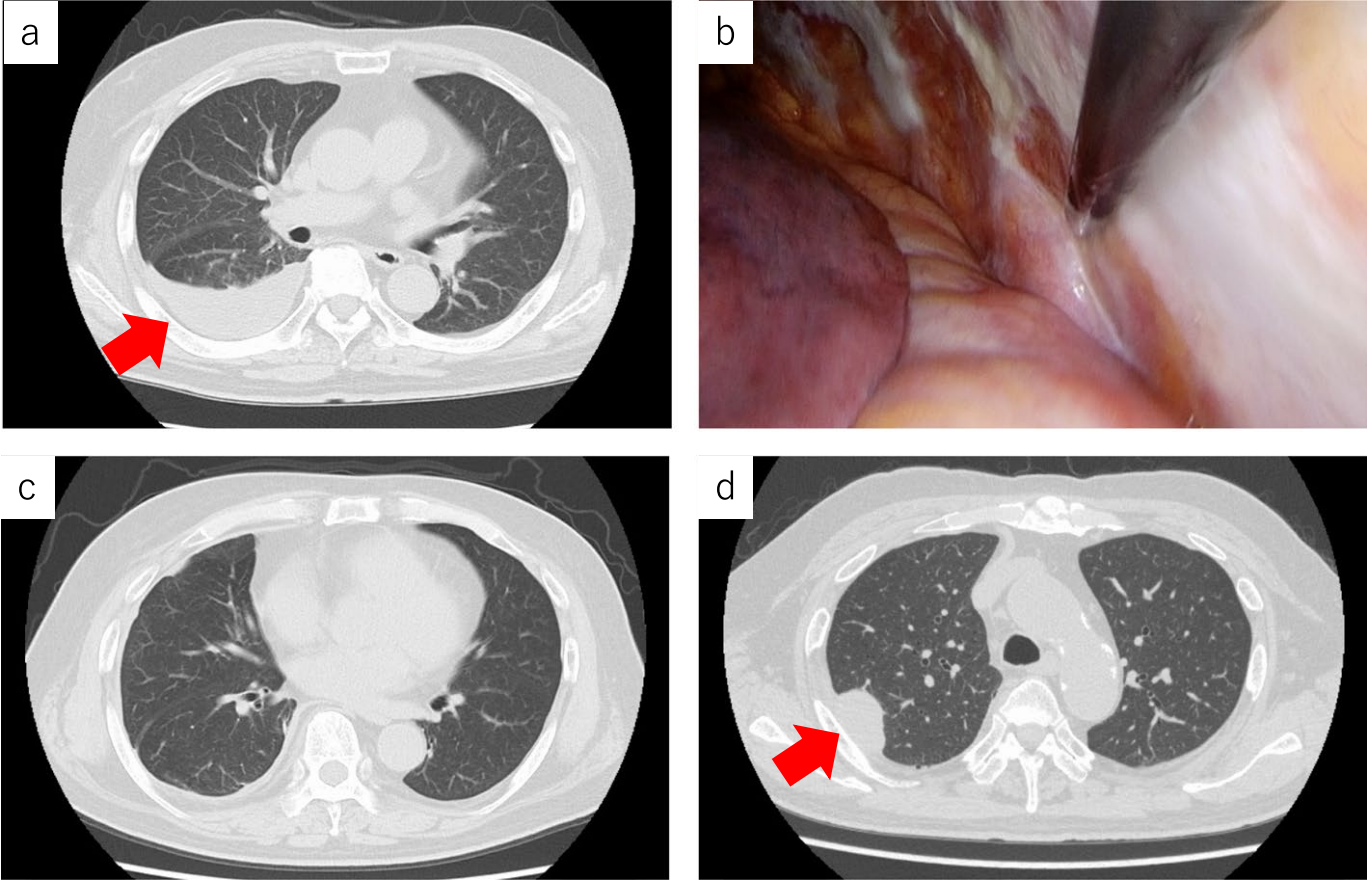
Clinical images. a Chest computed tomography showed a moderate amount of right pleural effusion with a slight bilateral pleural thickening. b A video-assisted thoracoscopic surgery biopsy of the right parietal pleura showed plaque-like pleural thickening, but no visible tumor. c The right pleural effusion decreased within five months from the first biopsy. d A nodular tumor approximately 3 cm in size appeared in the right pleura nine months from the first biopsy
